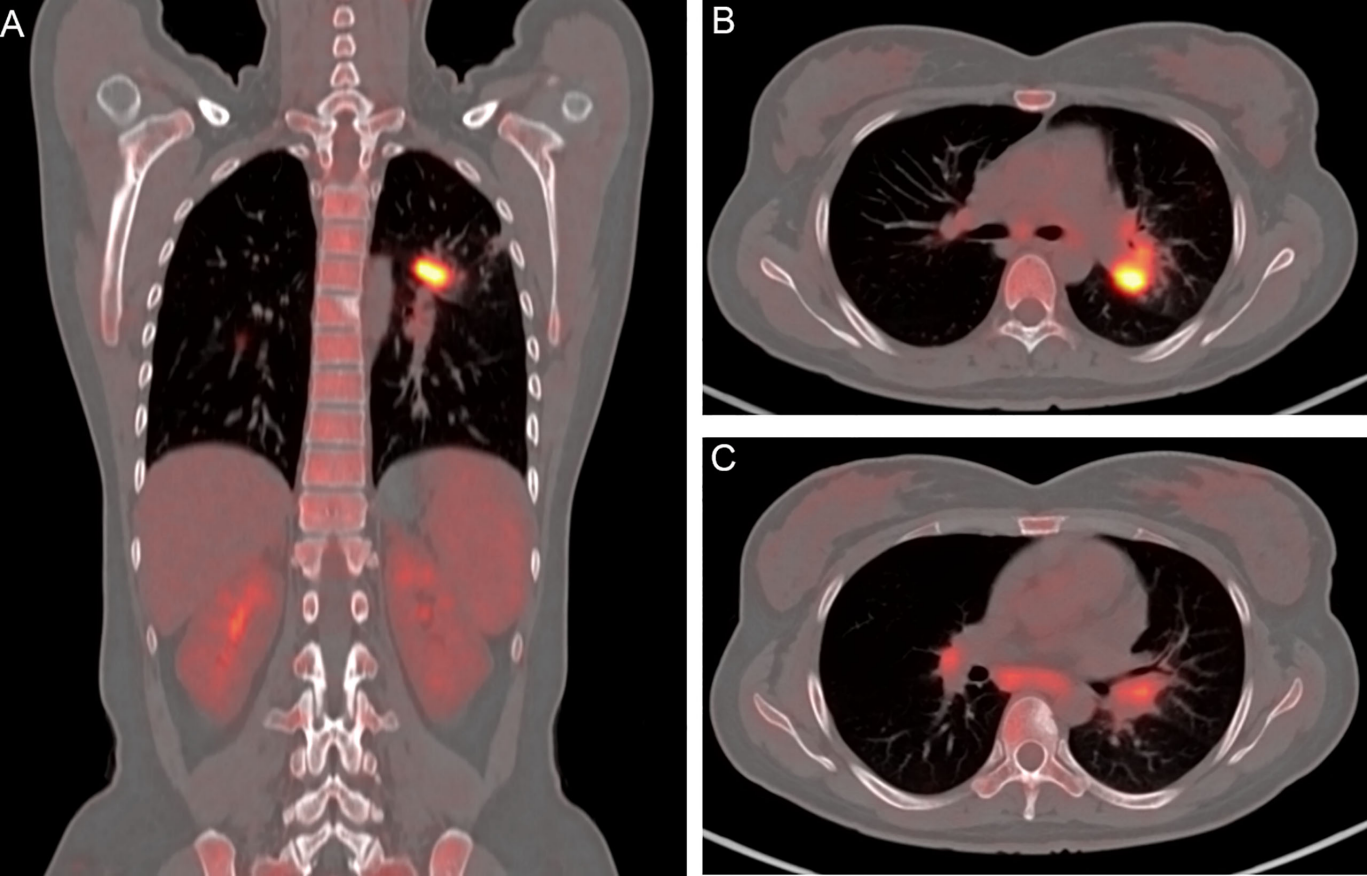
Positron emission tomography/X-ray computed tomography imaging results of the patient. (A) Thoracic (T-6) vertebral body showing low metabolism. (B) Left upper lobe of the lung showing high metabolism. (C) Osteolytic damage found in the vertebrae.
